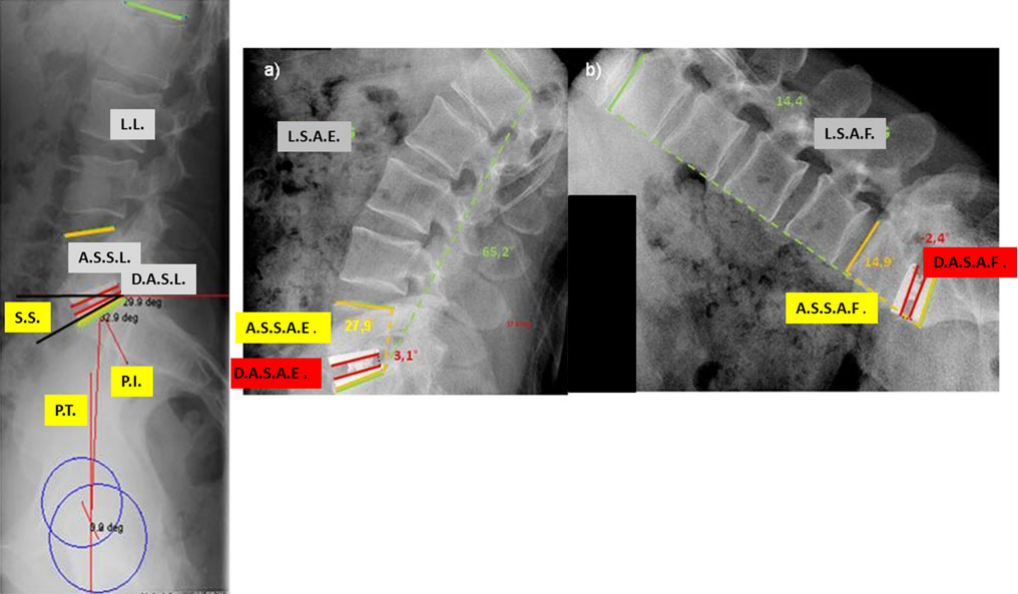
Measurement of lumbopelvic parameters and calculation of range of motion on flexion/extension X-rays. *L.L.* lumbar lordosis, *A.S.S.L.* adjacent segment segmental lordosis, *D.A.S.L.* disc arthroplasty segmental lordosis, *S.S.* sacral slope, *P.I.* pelvic incidence, *P.T.* pelvic tilt, *L.S.A.E.* lumbar sagittal angle for extension, *L.S.A.F.* lumbar sagittal angle for flexion, *A.S.S.A.E.* adjacent segment sagittal angle for extension, *A.S.S.A.F.* adjacent segment sagittal angle for flexion, *D.A.S.A.E.* disc arthroplasty sagittal angle for extension, *D.A.S.A.F.* disc arthroplasty sagittal angle for flexion: D.A.S.A.F. Intervertebral flexion–extension angle is calculated according to Wong [40, 41]: (D.A.S.A.E)–(D.A.S.A.F.) for the arthroplasty level. (A.S.S.A.E.)–(A.S.S.A.F.) for the adjacent level
